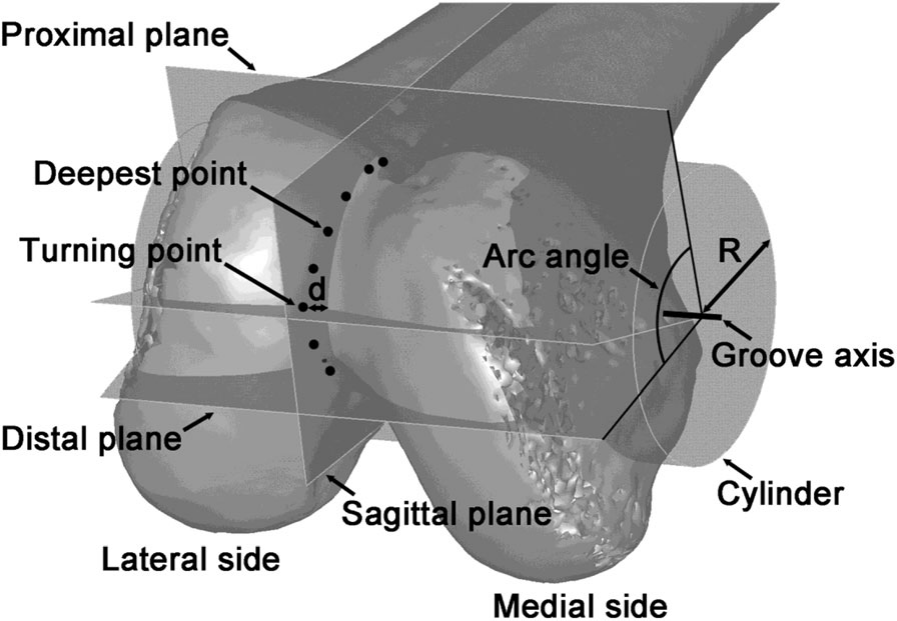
Methodology of classifying the trochlear groove tracking in terms of morphology. Three planes were established in three-dimensional models of the knee, constructed based on computed tomography data. A cylinder with its axis parallel to both the coronal and transverse planes was defined, and its radius was adjusted to allow the cylindrical surface to closely fit the trochlear groove. Coaxial cutting planes rotating in 15° increments (from 45° to 90°) about the trochlear groove axis (from the proximal to distal point) were defined. Subsequently, the deepest point of the trochlear groove was marked in each cross section. The distance from the turning point of the trochlear groove to the mechanical axis was measured (represented by d)
